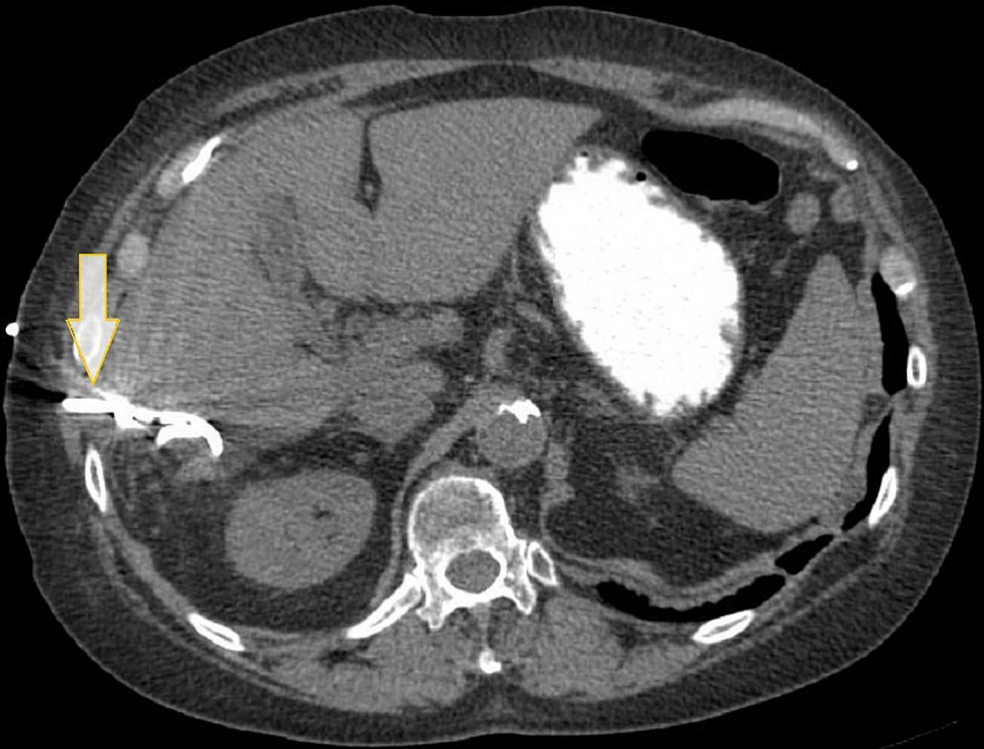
Follow-up computed tomography scan of the abdomen and pelvis without contrast shows almost complete resolution of the biloma. The arrow shows a subhepatic pigtail drain.
